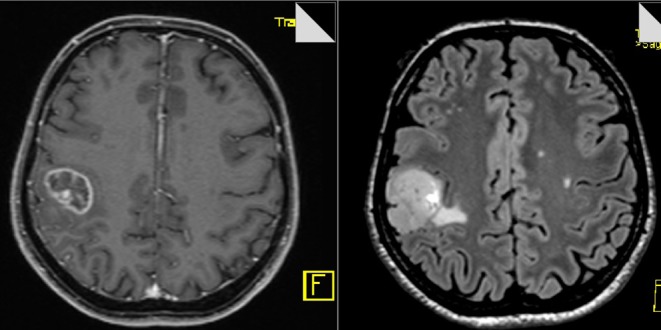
Pre-operative MR image (T1-weighted and FLAIR sequences, axial planes) showing circular contrast-enhancement and moderate edema.
